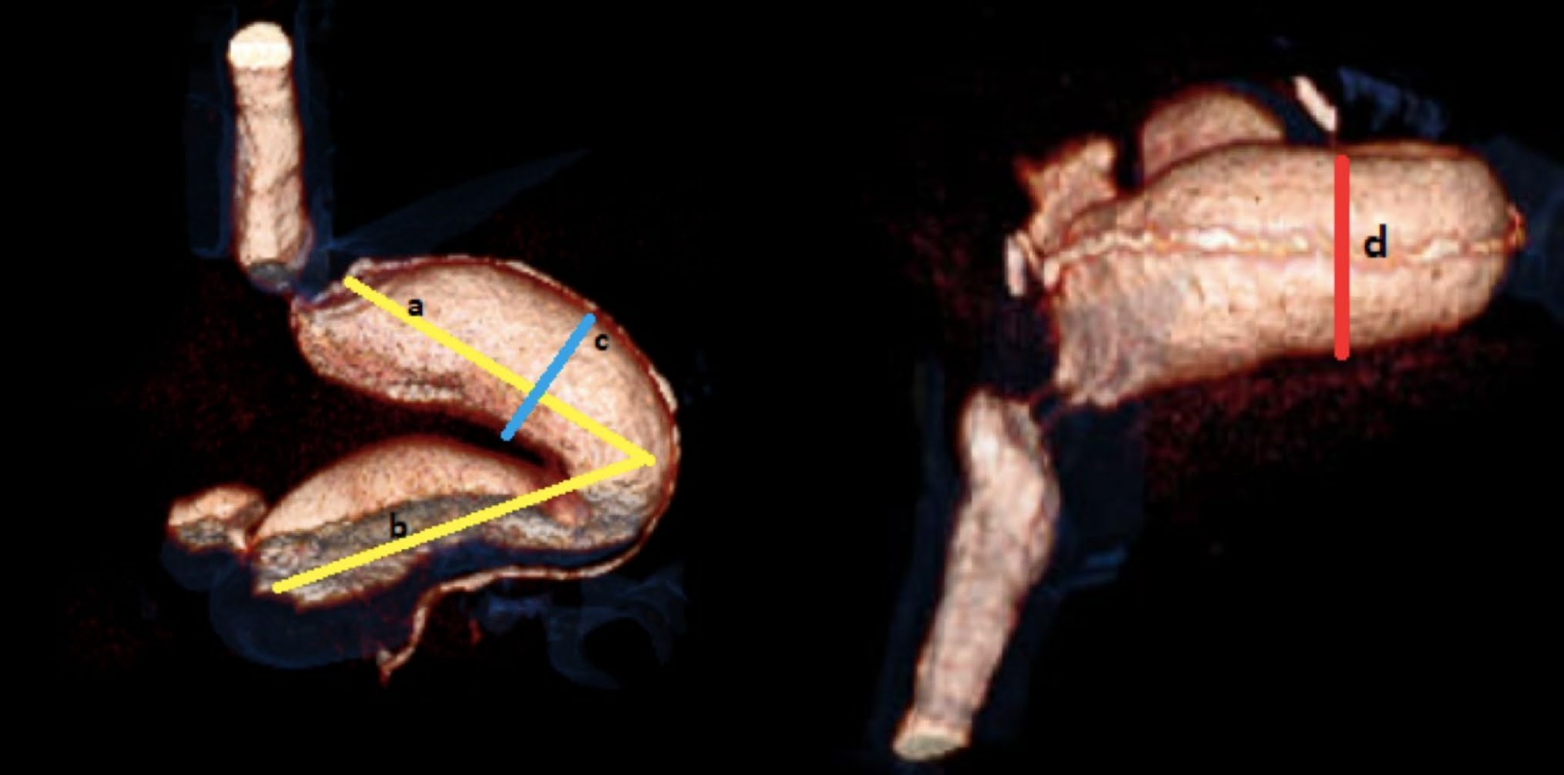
Dimensional computed tomography image of gastric volume calculation
